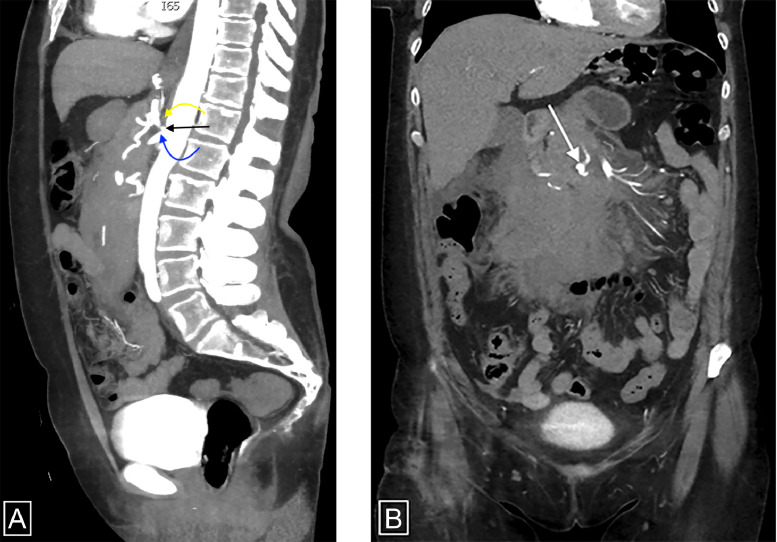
(A) Sagittal CT angiography of the abdomen and pelvis reveals compression of the celiac artery (black arrow) by the median arcuate ligament (curved yellow arrow), resulting in characteristic narrowing with a “hooked” appearance. The superior mesenteric artery (SMA) is shown (curved blue arrow) (B) Coronal CT angiography of the abdomen and pelvis reveals 6mm Arc of Bühler pseudoaneurysm (white arrow) surrounding by extensive acute mesenteric hemorrhage.
